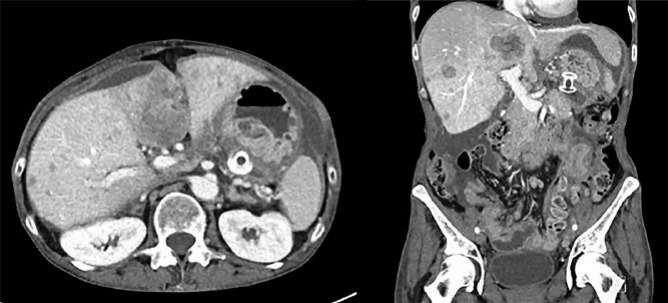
Axial and coronal abdominal CT images 3 months after EUS-guided transgastric placement of LAMS. ESU, endoscopic ultrasound.
